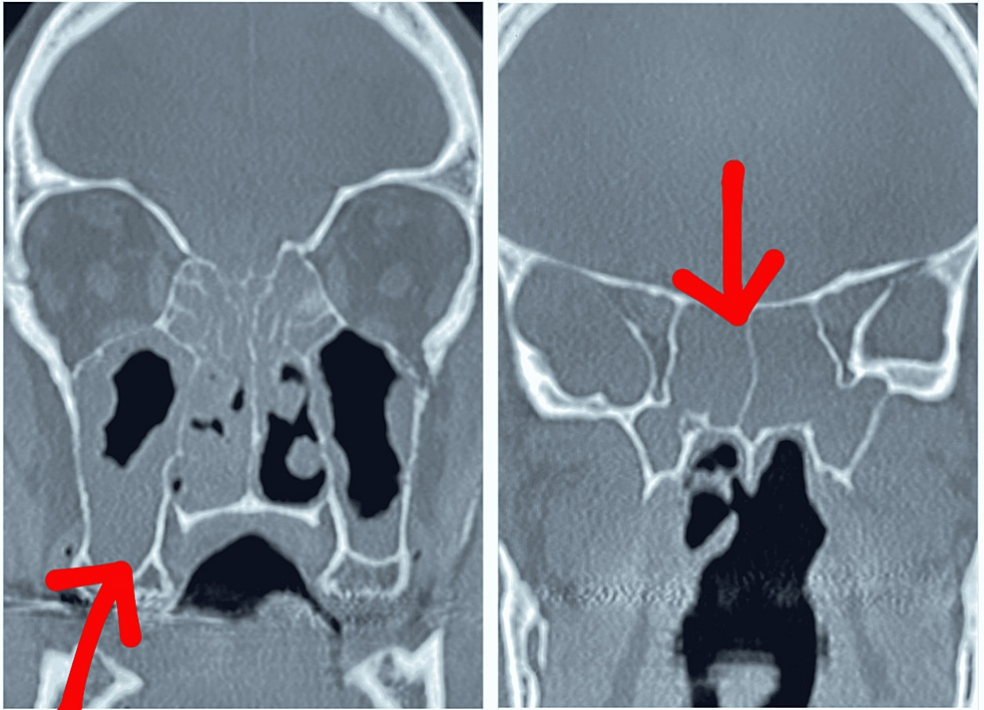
Pansinusitis seen on CT imaging (red arrows)
